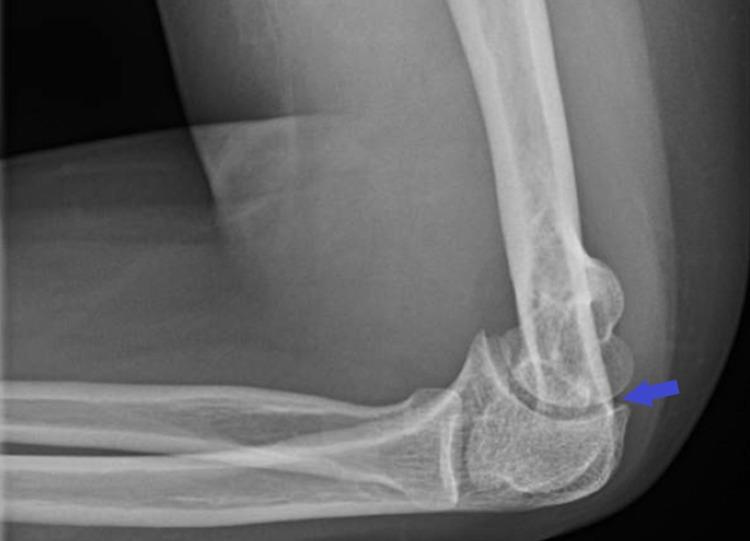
Radiography of the left elbow showing joint effusion (blue arrow)
